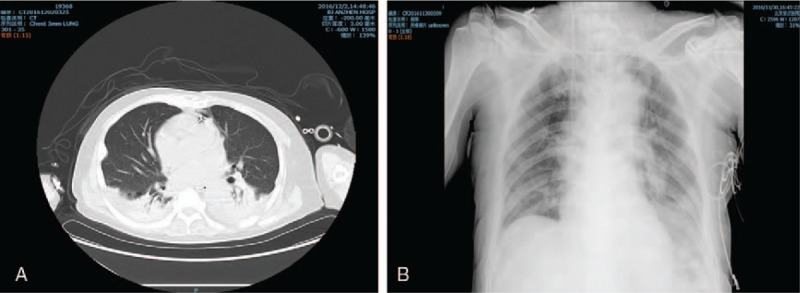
(A) The postoperative chest CT scan, and (B) the postoperative bedside chest radiograph. There was evidently exudative lesion and lung consolidation in bilateral lung tissue.
